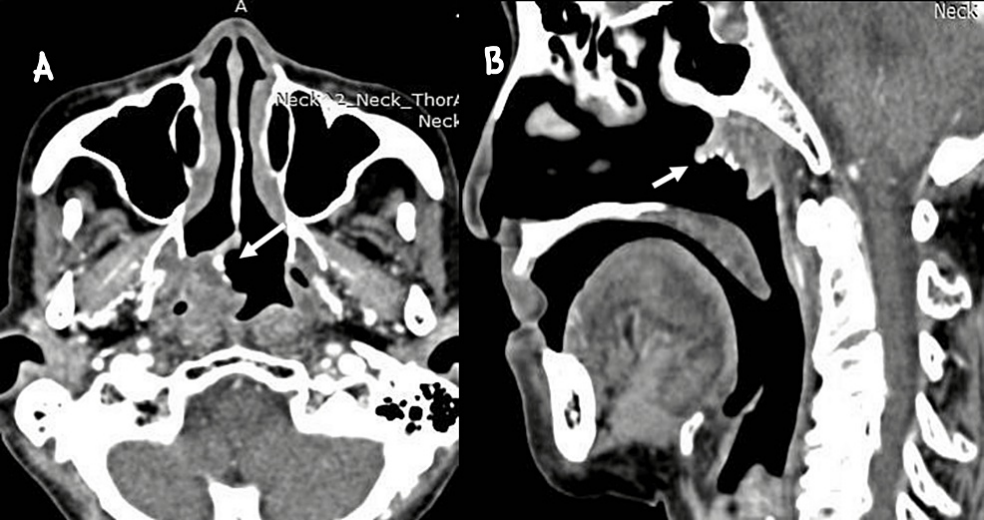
(A) Axial cut of CT scan showed tumour arising from the right nasopharynx with calcification within it (white arrow); (B) Sagittal cut of CT scan showed irregular tumour at the roof of nasopharynx with calcification within it (white arrow)
